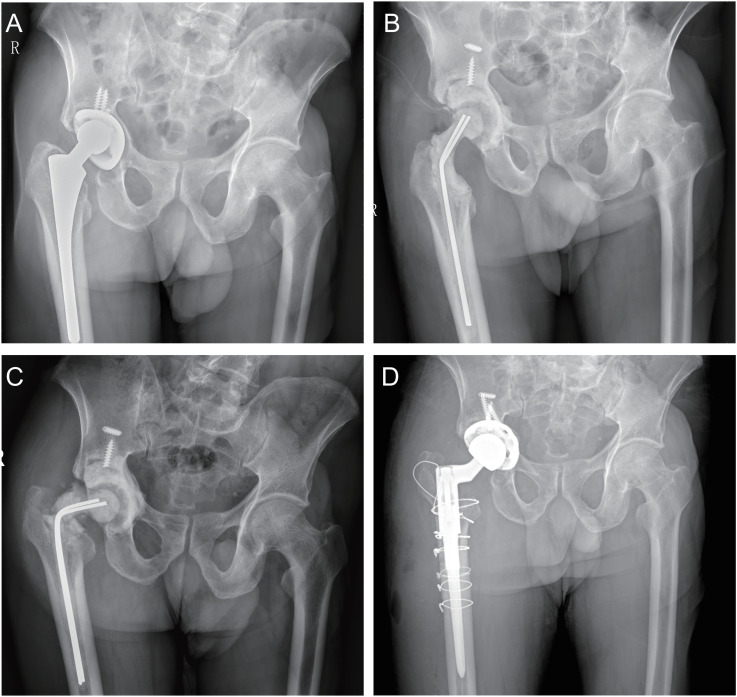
(A) postoperative anteroposterior pelvic radiograph; (B) anteroposterior pelvic radiograph 3 days after cement spacer implantation; (C) anteroposterior pelvic radiograph showing spacer fracture; (D) anteroposterior pelvic radiograph 2 years after two-stage revision.
